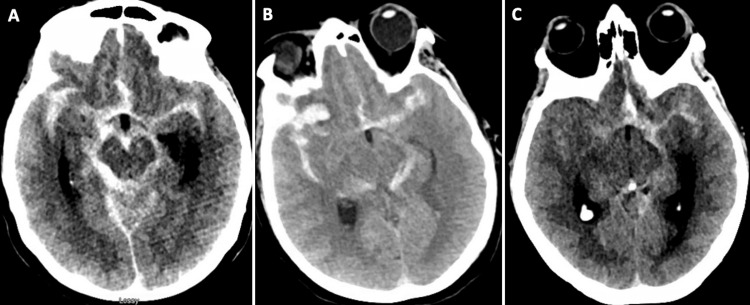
Initial non-contrast CT brain of patient 1 (A), patient 2 (B), and patient 3 (C) demonstrating diffuse subarachnoid hemorrhage
